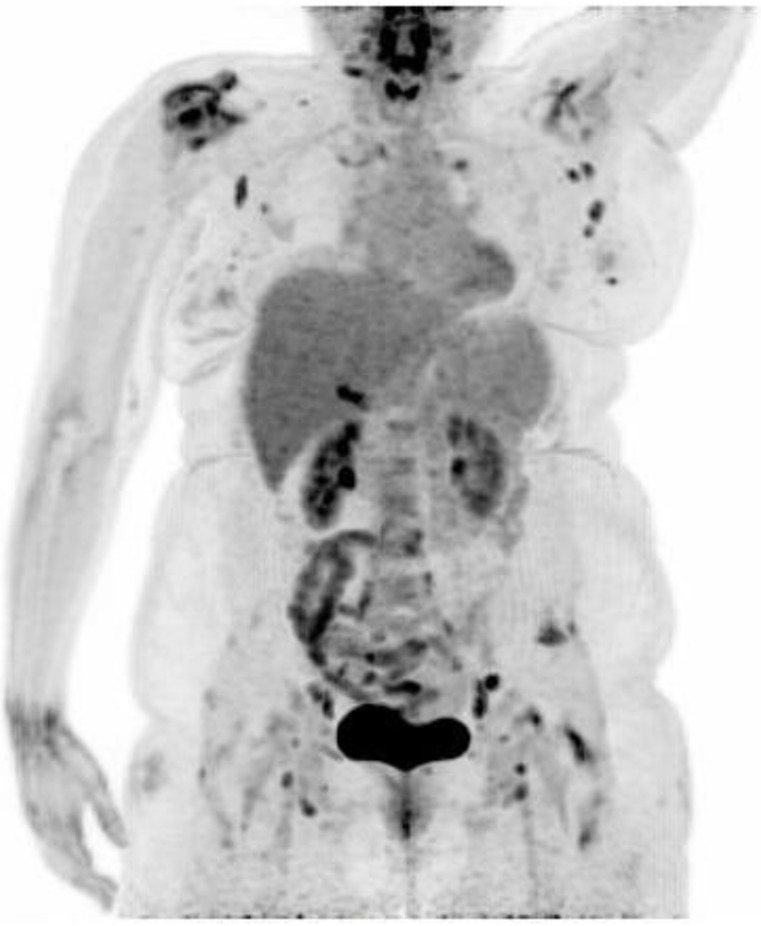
A 50-year old woman of Indian descent living in Denmark, with a prior history of knee arthrosis, presented with fever, myalgia and arthralgia which had developed during a return visit to New Delhi, India. Two weeks after returning to Denmark the generalised arthralgia and myalgia persisted to a degree where the patient was mainly confined to her bed. The symptoms were accompanied by extreme fatigue and fever sensation. When examined, the patient’s upper extremities were almost immobile due to soreness of shoulder, elbow and wrist joints, as well as reduced mobility of lower extremities, with slight edema of both feet. On inspection there were no visible signs of joint inflammation or skin rash. On admission we found elevated a sedimentation rate of 51 mm (normal range: 2–20 mm), C-reactive protein 25 mg/L (normal range: 0–10 mg/L), d-dimer 1, 9 FEU (fibrinogen equivalent units)/L (normal range < 0.5 FEU/L) and slightly elevated Lactate dehydrogenase at 212 U/L (normal range: 105–205 U/L). White blood cell count, creatinin kinase, antineutrophil cytoplasmic antibody, and antinuclear antibody were in the normal range. Dengue IgG and IgM were negative. NSAID treatment in combination with tramadole was initiated with no effect on the patient’s severe arthralgia. An ^18^F-FDG PET/CT (fluorine-18 fluoro-2-deoxy-d-glucose positron emission tomography/computed tomography) was performed four weeks after debut of symptoms. Due to severe pain the patient was not able to place her right arm over her head during the scanning. The scan revealed pathological ^18^F-FDG uptake in enlarged lymph nodes on both side of the diaphragm, inflammation of both shoulder and hip joints, as well as ^18^F-FDG uptake in a nodule in the left breast. The main differential diagnoses were reactive arthritis, polymyalgia rheumatica, lymphoma or paraneoplastic phenomena. In response to these findings prednisolone 25 mg daily was initiated, which significantly relieved the patient’s symptoms within days. Concurrently, the patient was referred for further evaluation of the PET positive breast nodule, rheumatological evaluation, and a lymph node biopsy was considered.
